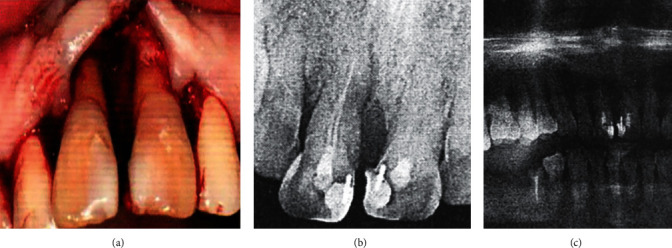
(a) The photo of the patient at the time of operation; (b) the apical sheet; and (c) the X-ray film at the initial diagnosis.
